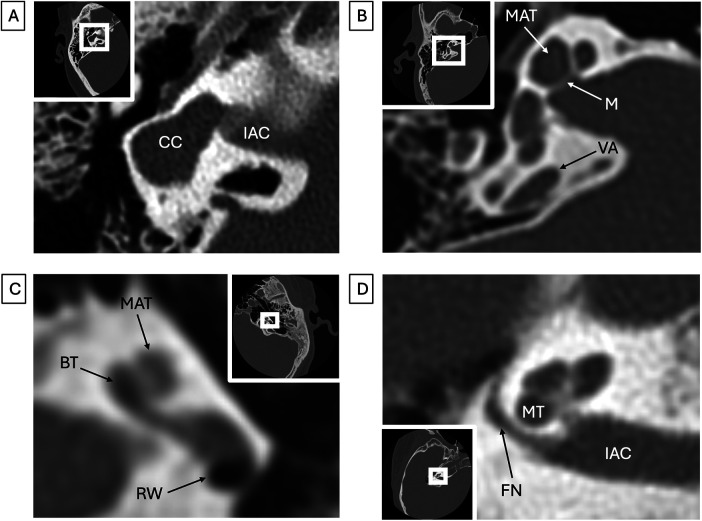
Inner ear congenital abnormalities (all images represent different patients). (A) Common cavity malformation. Axial CT shows a common cavity (CC) comprising both the vestibule and cochlea, which communicates with a dysplastic enlarged internal auditory canal (IAC). (B) Incomplete partition (IP). Axial CT in a patient with IP type II shows coalescence of the middle and apical turns (MAT), a deficient modiolus (M), and an enlarged vestibular aqueduct (VA). Round window (RW) is shown for reference. (C) Cochlear hypoplasia. Axial CT in a patient with branchio-oto-renal syndrome shows a dysplastic “unwound” basal turn (BT) and hypoplasia of the apical and middle turns (MAT). (D) Aberrant facial nerve course. Axial CT shows aberrant course of the labyrinthine segment of the facial nerve (FN) extending posterior to the middle turn of the cochlea (MT) in a patient with CHARGE syndrome. Internal auditory canal (IAC) is shown for reference.
